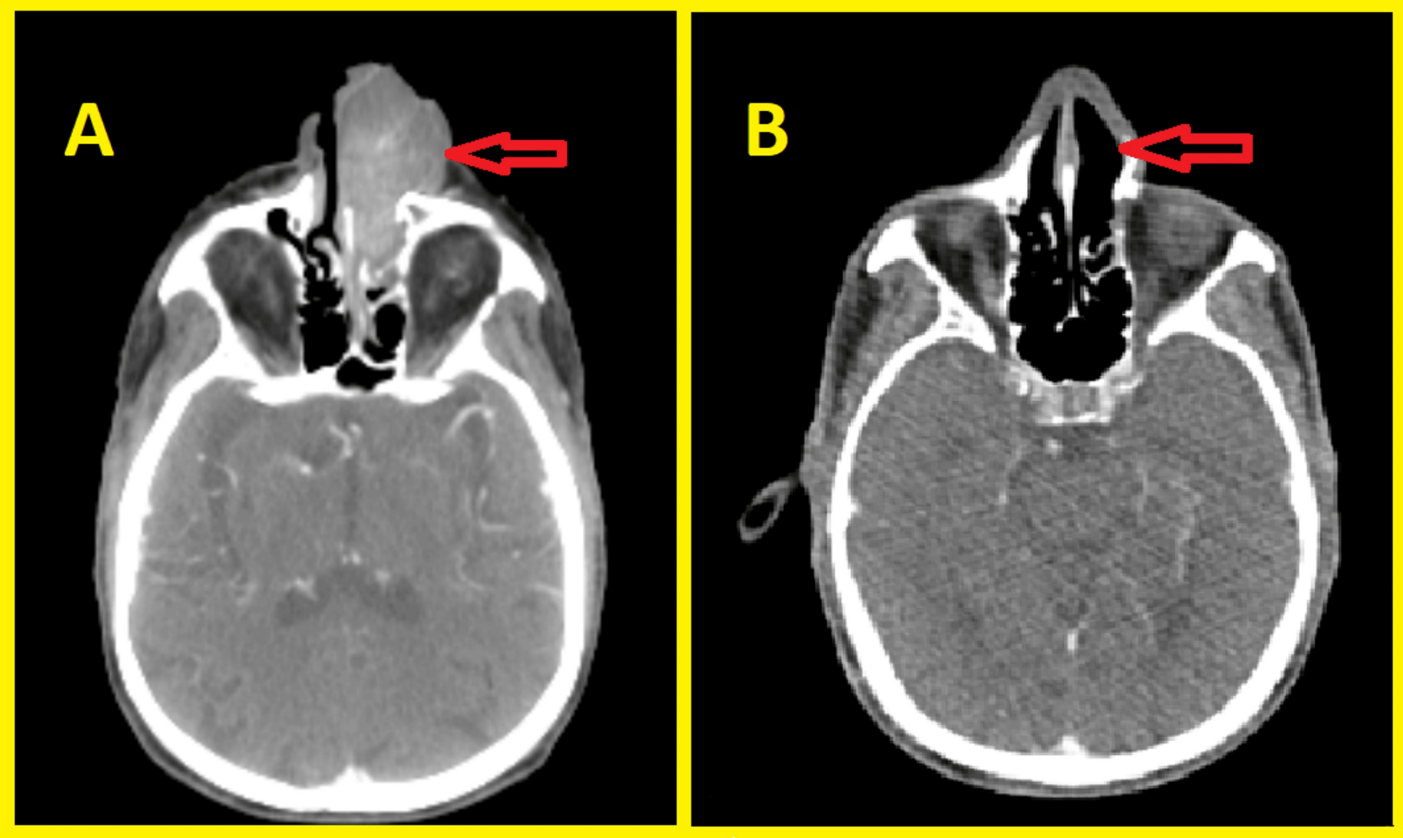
CT scan showing the nasal process before (A) and nine months after the completion of radiation therapy (B) (red arrows).
